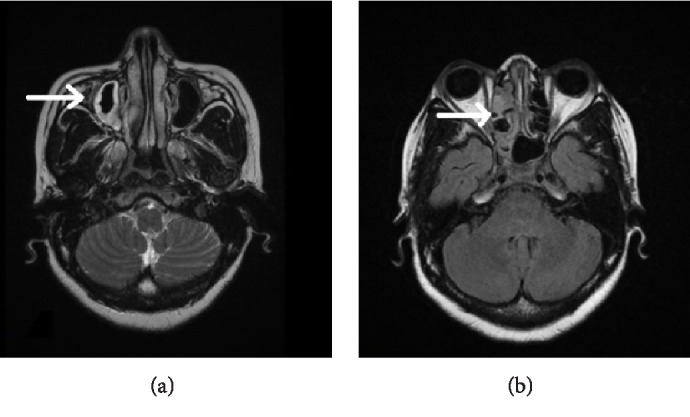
Case 1, magnetic resonance with right ethmoid and sphenoid occupation with increased compared to previous CT (Figure 2).
